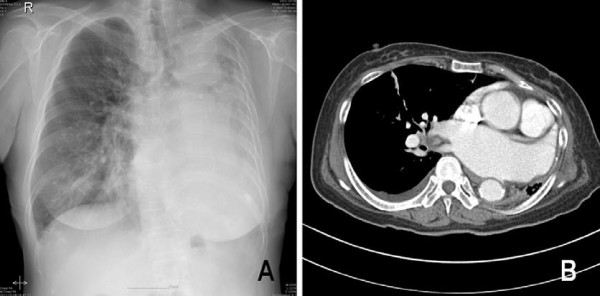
Images of the patient’s chest prior to surgery. (A) Preoperative chest X-ray and (B) enhanced computed tomographic scan with axial image.
